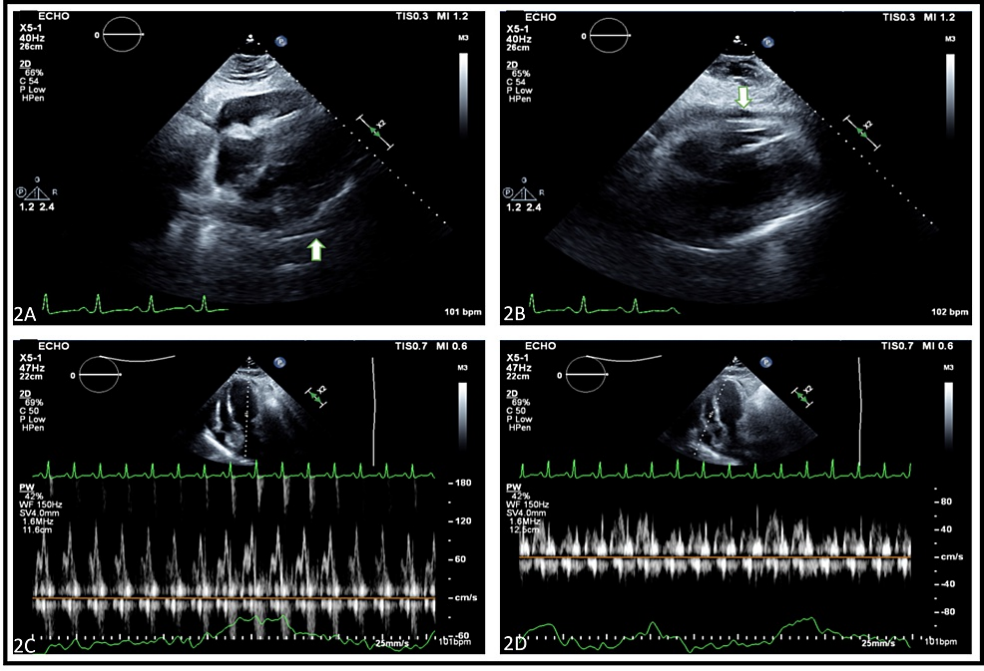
Transthoracic echocardiogram at presentation demonstrating moderate anteriorly location pericardial effusion (2A and 2B) without evidence of significant respiratory variation of inflow velocities across the mitral (2C) and tricuspid (2D) valves
